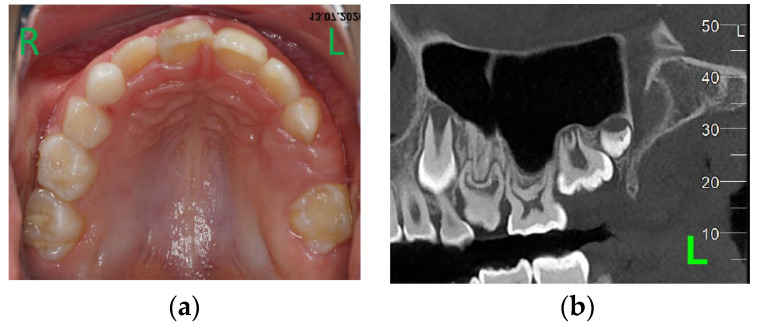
Age of 9 y and 7 m. (a) Occlusal view of the upper arch; (b) CBCT confirmed agenesis of 24, 25, and impaction of 64.
